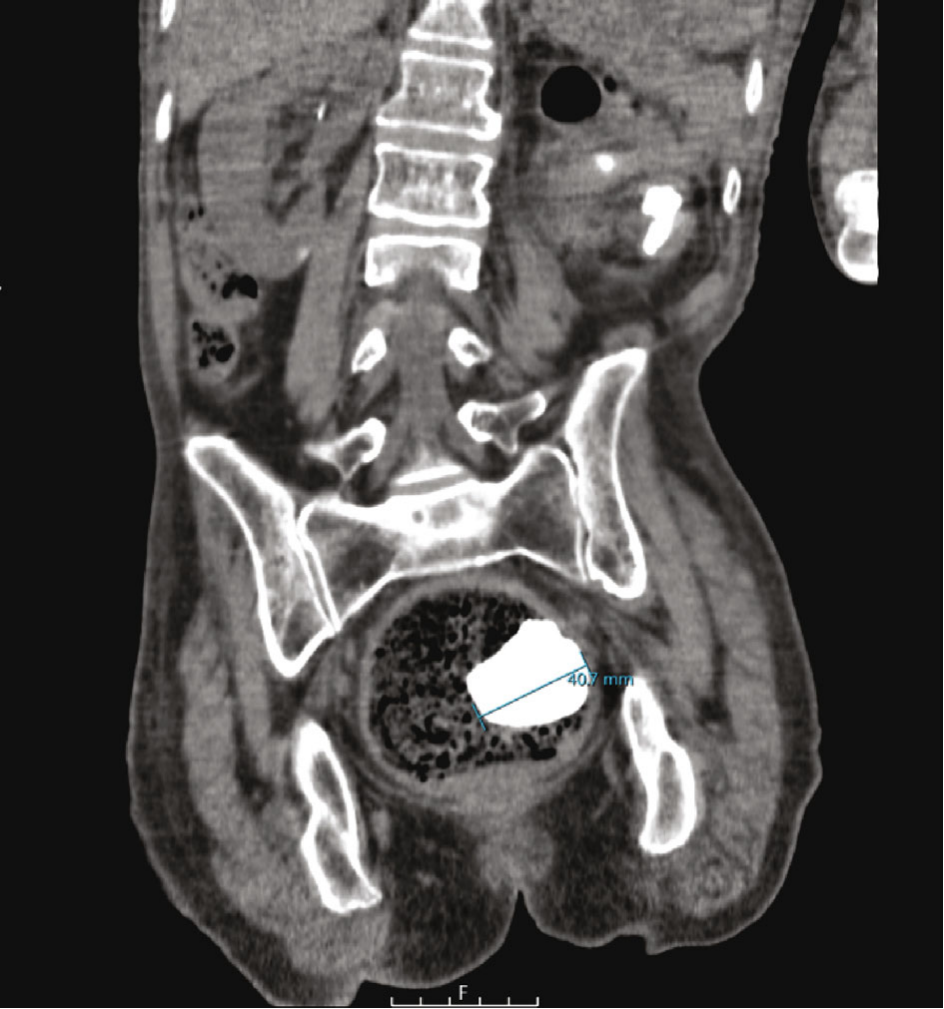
Repeat CT KUB scan showing the staghorn calculus, previously visualized in the fistula, now located inside the rectum.
